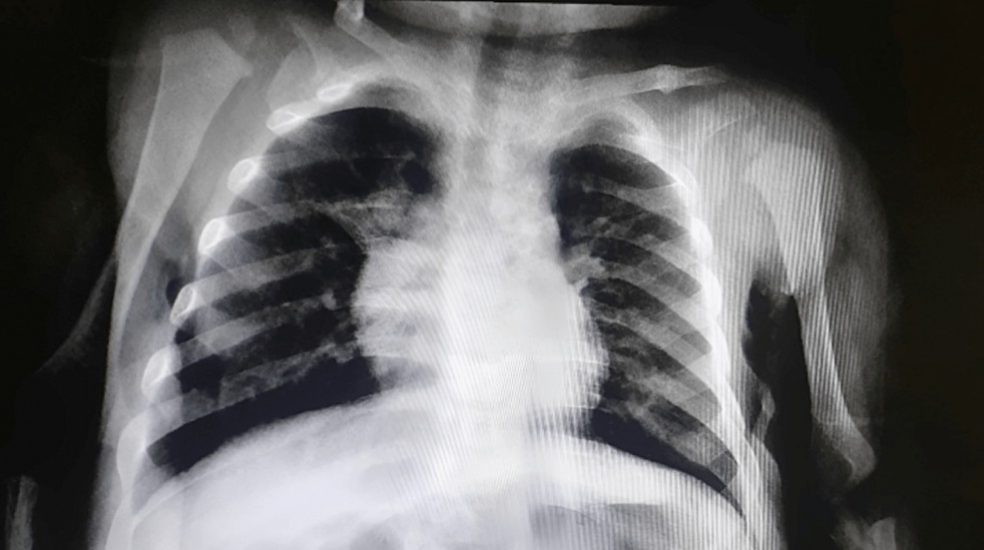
Chest X-ray of the patient. Bilateral lung infiltrations with increased vascular markings.
